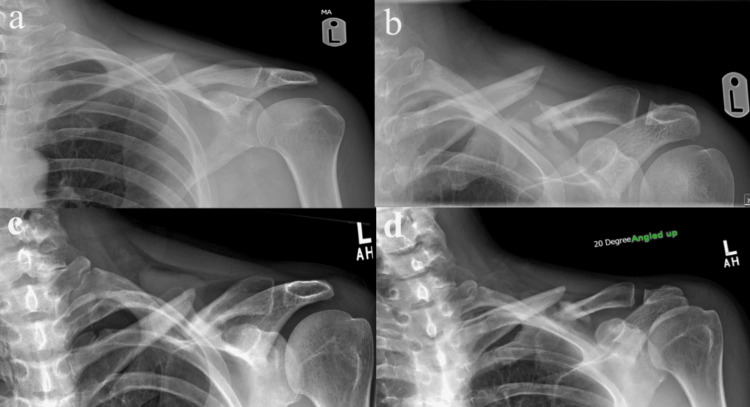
Case 2: Left shoulder X-ray, including initial AP (a) and cephalic tilt (b), revealing significant displacement and shortening, and six-week (three months) (c and d) follow-up X-ray indicating nonunion AP: anterior-posterior
